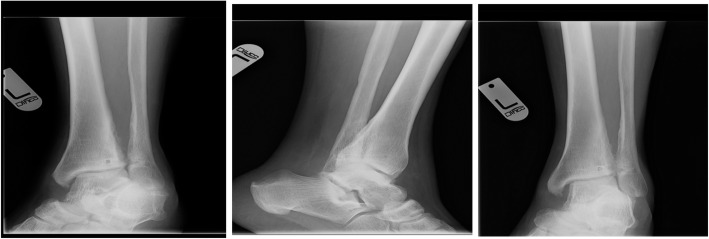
Postoperative radiographs at 13 months. An internal rotation (mortise), lateral and anterior-posterior view views of the left ankle (*left to right* respectively). The radiographs seem to exhibit normal syndesmosis appearance and position
